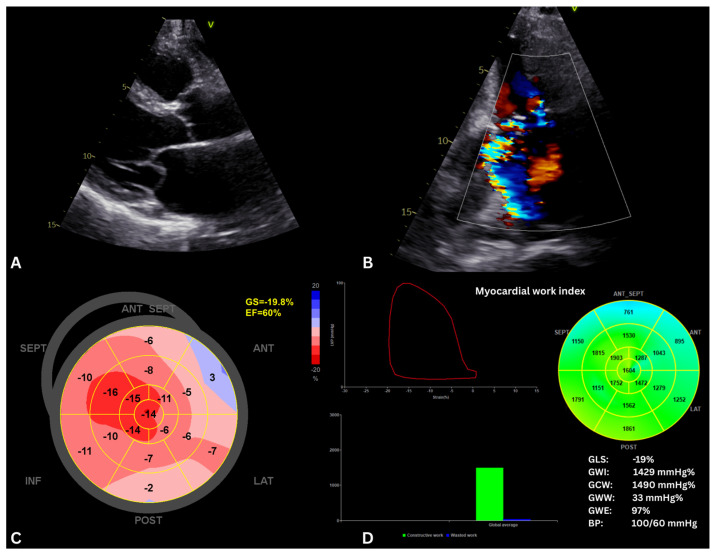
Myocardial work analysis in a patient with severe mitral regurgitation. (A) Parasternal long-axis view showing posterior mitral valve prolapse. (B) Apical 2-chamber view with color Doppler demonstrating severe mitral regurgitation with posteriorly directed jet. (C) Speckle-tracking analysis identifying preserved LV function (GLS −19.8%, LVEF 60%). (D) Myocardial work analysis showing preserved GWI, GCW and GWE, but very low wasted work (GWW = 33 mmHg%), suggesting minimal energy loss. Patients with extremely low GWW, even with preserved LVEF and GLS, may be at higher risk of adverse outcomes [38], including progression of heart failure or complications from severe mitral regurgitation. In this context, MW could help identify patients who might benefit from earlier or more aggressive management, such as closer follow-up, optimization of afterload-reducing therapy, or consideration for mitral valve intervention. In the panel (C), red shades indicate negative strain values, while blue indicates positive strain values. In panel (D), the myocardial work bull’s-eye shows green for normal/constructive myocardial work and blue for negative/wasted work. GS/GLS, global longitudinal strain; EF, ejection fraction; GWI, global work index; GCW, global constructive work; GWW, global wasted work; GWE, global work efficiency; BP, blood pressure; V, apical view orientation marker.
